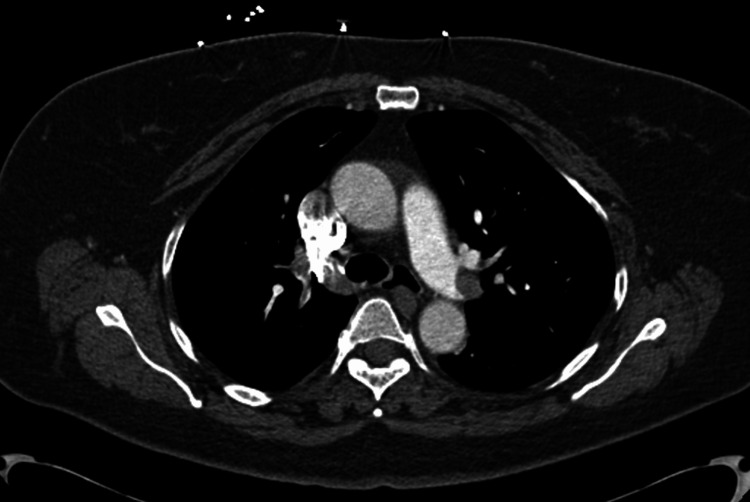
A computed tomography angiogram of the chest (axial images) shows a left-sided pulmonary embolism.
